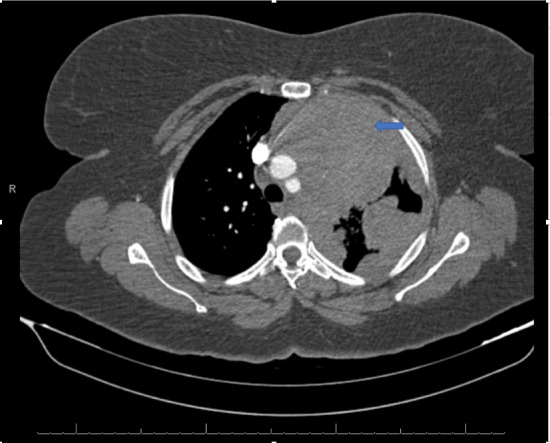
The computed tomography scan of the chest showed a large
